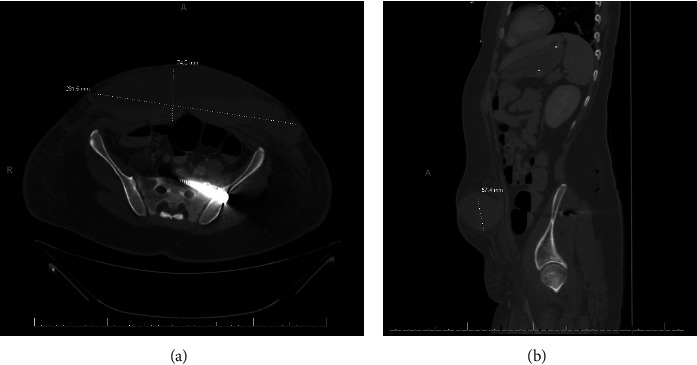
Patient imaging demonstrating an aspect of the patient's polytrauma injuries involving a ML lesion: (a) CT imaging of axial view of Morel-Lavallée hematoma measuring 74 mm × 281.5 mm; (b) sagittal view of CT imaging of Morel-Lavallée hematoma measuring 57.4 mm in height.
